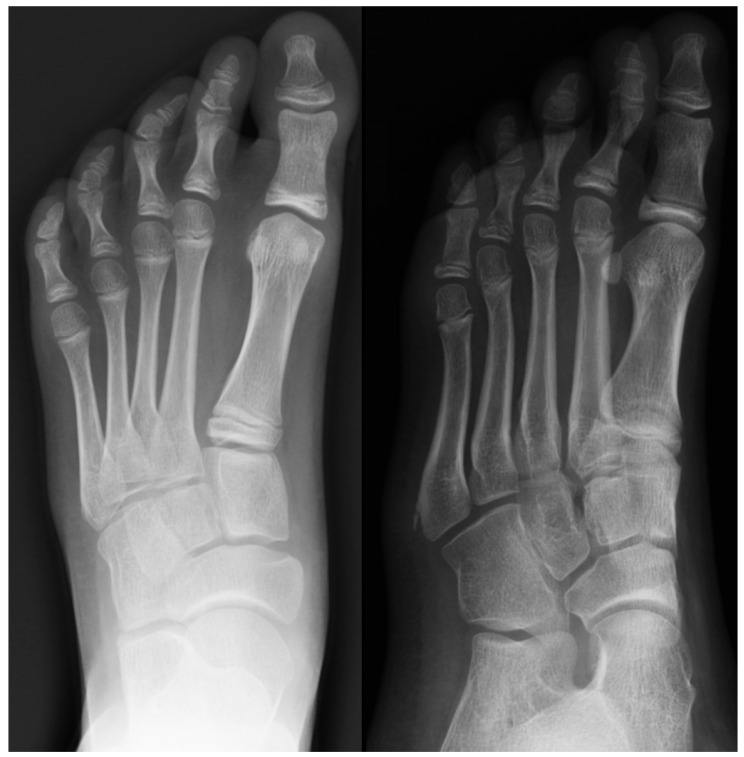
Radiograph (especially on oblique view) of an 8-year-old male patient showing fragmentation of the lateral apophysis of the fifth metatarsal base, in the absence of trauma.
